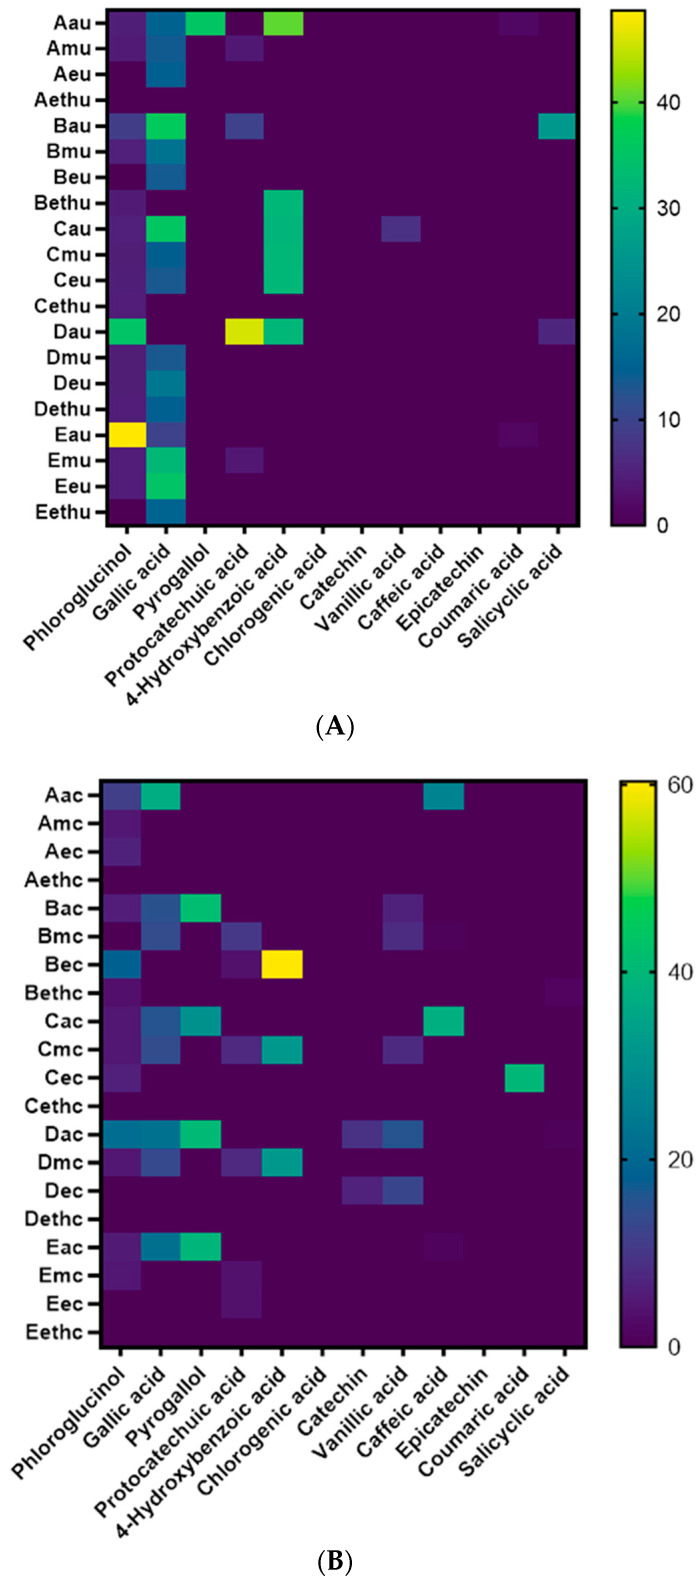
(A) Heatmap showing phenolic compounds’ distribution and concentration among five seaweed species extracted in four different solvents via ultrasonication extraction. (B) Heatmap showing phenolic compounds’ distribution and concentration among five seaweed species extracted in four different solvents via conventional extraction.
